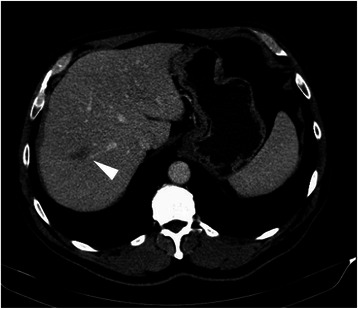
Contrast enhanced CT after 12 cycles of oxaliplatin-based adjuvant chemotherapy showing a new liver nodule in segment 8, in close proximity to the right hepatic vein (arrowhead).
